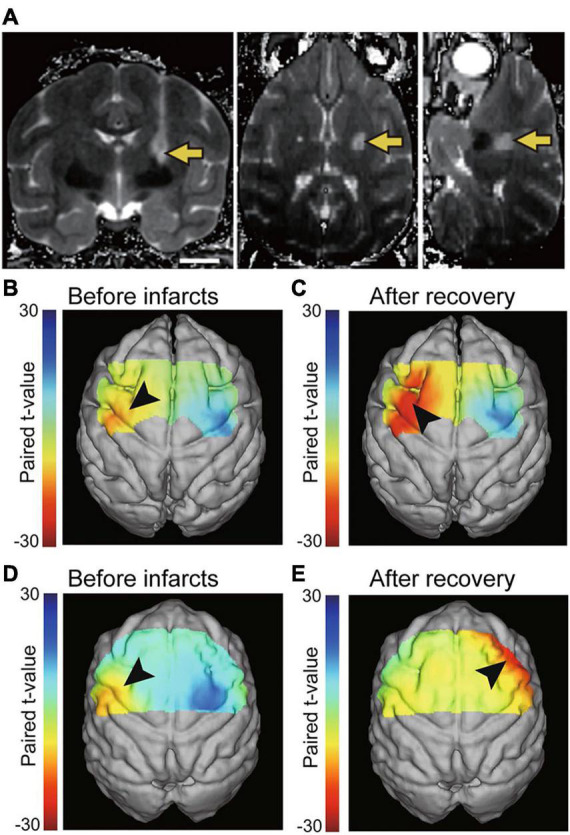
(A) T2-weighted MRI showing the location of infarcts in the posterior internal capsule one day after endothelin-1 injection (coronal, axial, and sagittal images). The arrows indicate the infarct. Scale bars = 10 mm. Reproduced from Figure 1 of the study by Murata and Higo (2016). (B–E) Brain activation during voluntary hand movements before infarcts (B,D) and after motor recovery from the internal capsular infarcts. Before infarcts, focal activation was observed in the hand area of the primary motor cortex (arrowhead in B,D). After motor recovery, increased activation of the premotor area was identified (arrowhead in C,E). The cortex contralateral to the stroke plays a greater role in recovery when lesions are more severe (E). Reproduced from Figures 2, 4 of the study by Kato et al. (2020b).
